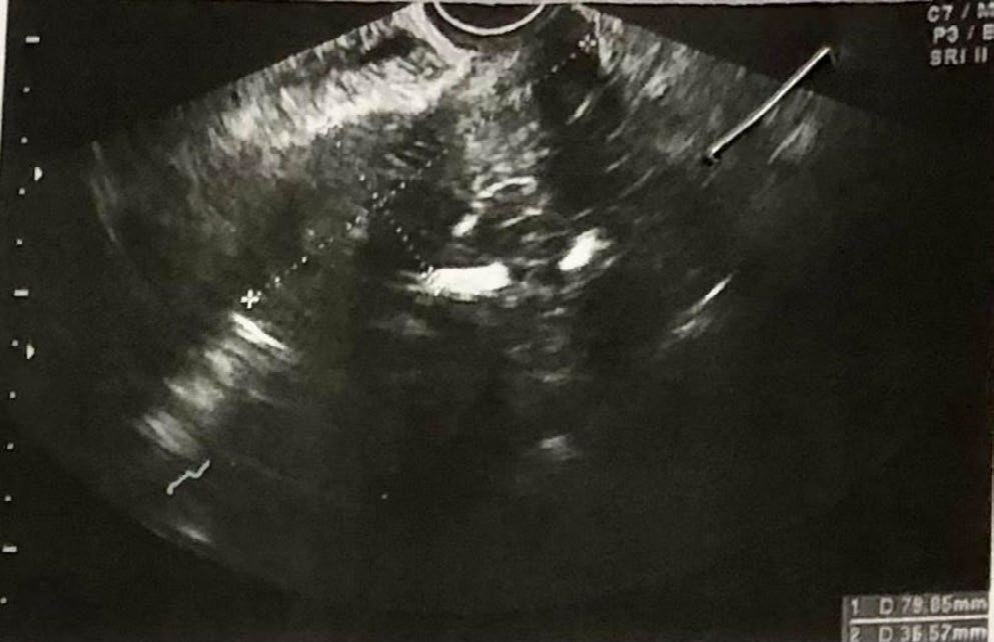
Ultrasound examination of the uterus and adnexa (abdominal).
